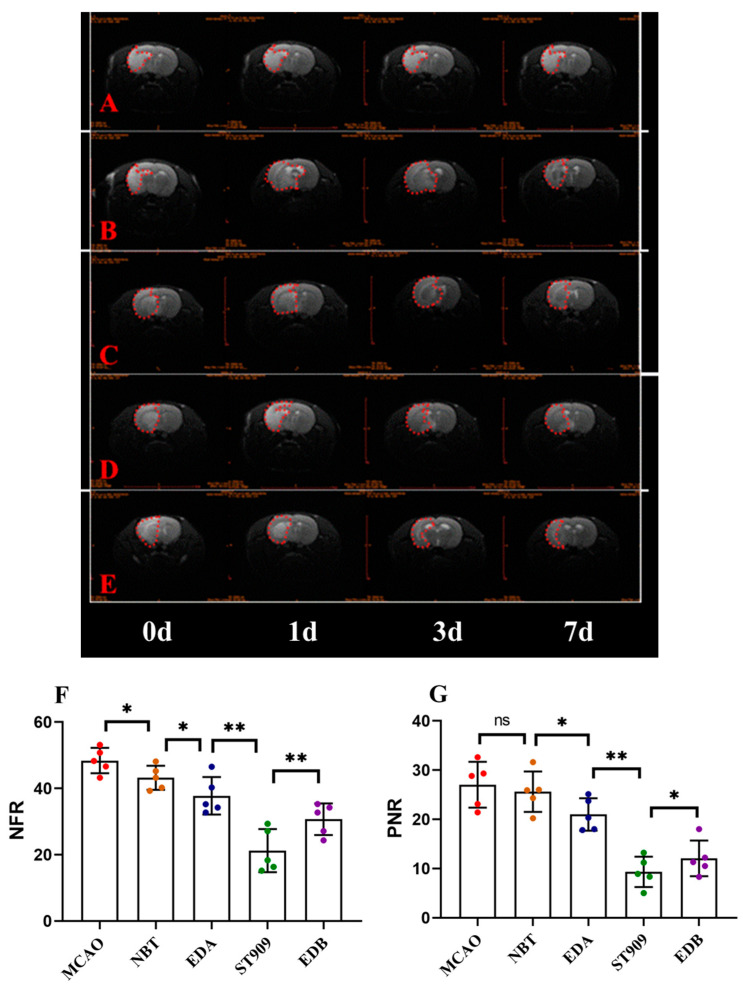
Representative Brain MRI images (A) MCAO; (B) NBT; (C) EDA; (D) EDB; (E) ST909 and relative apparent diffusion coefficient (rADC) value comparison (7 days) (F) NFR; (G) PNR. The unlabeled version is shown in Figure S3. One-way ANOVA test in (F,G), *n* = 5; the results as shown as mean ± S.D. , *p* < 0.01; *, *p* < 0.05; ns, *p* > 0.05). The red dotted area represents the brain edema area. The normalized functional recovery (NFR) index reflects the recovery of the lesion area relative to the normal area, while the penumbra-to-normal ratio (PNR) reflects the recovery of the penumbra relative to the normal area. The apparent hemispheric asymmetry observed in MRI images results from pathological cerebral edema secondary to ischemic injury.
